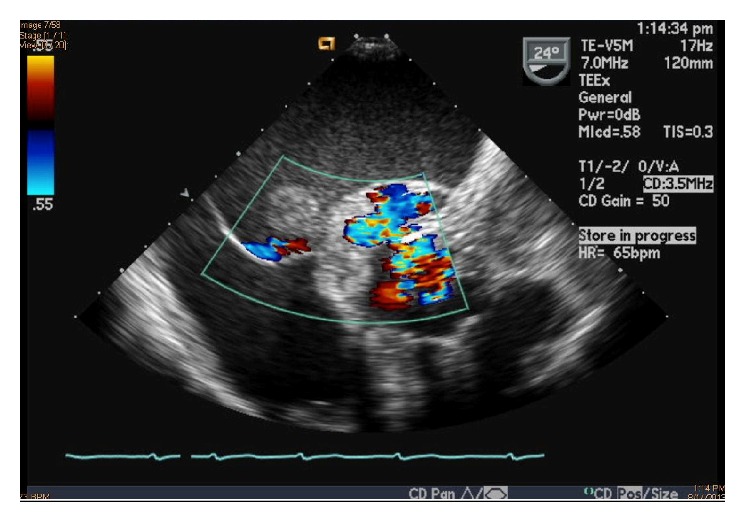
Transesophageal echocardiogram in short axis view with color flow Doppler showing blood flow from the bioprosthetic aortic valve, into the aortic root abscess, which has ruptured into the left atrium, forming an aortico-left atrial fistula.
